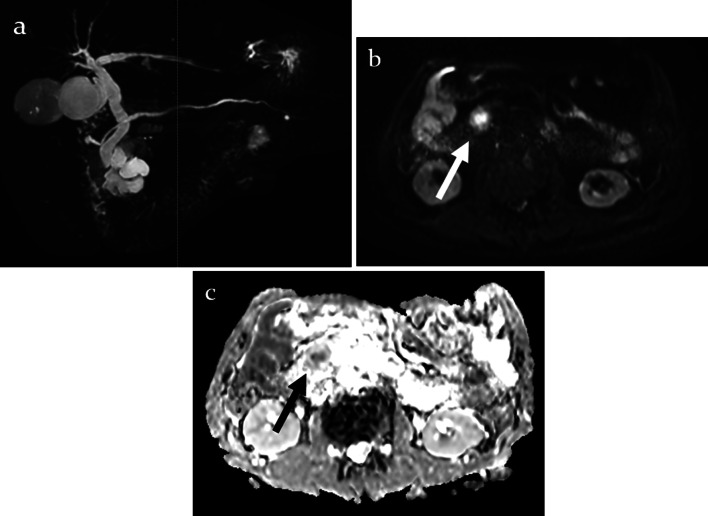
a Magnetic resonance cholangiopancreatography (MRCP) showed a multilobular cyst in the branch duct with communication between the cystic lesion and the main pancreatic duct. b Diffusion-weighted imaging (DWI) showed an area of hyperintensity in the mural nodule (white arrow). c Apparent diffusion coefficient (ADC) was decreased (black arrow)
